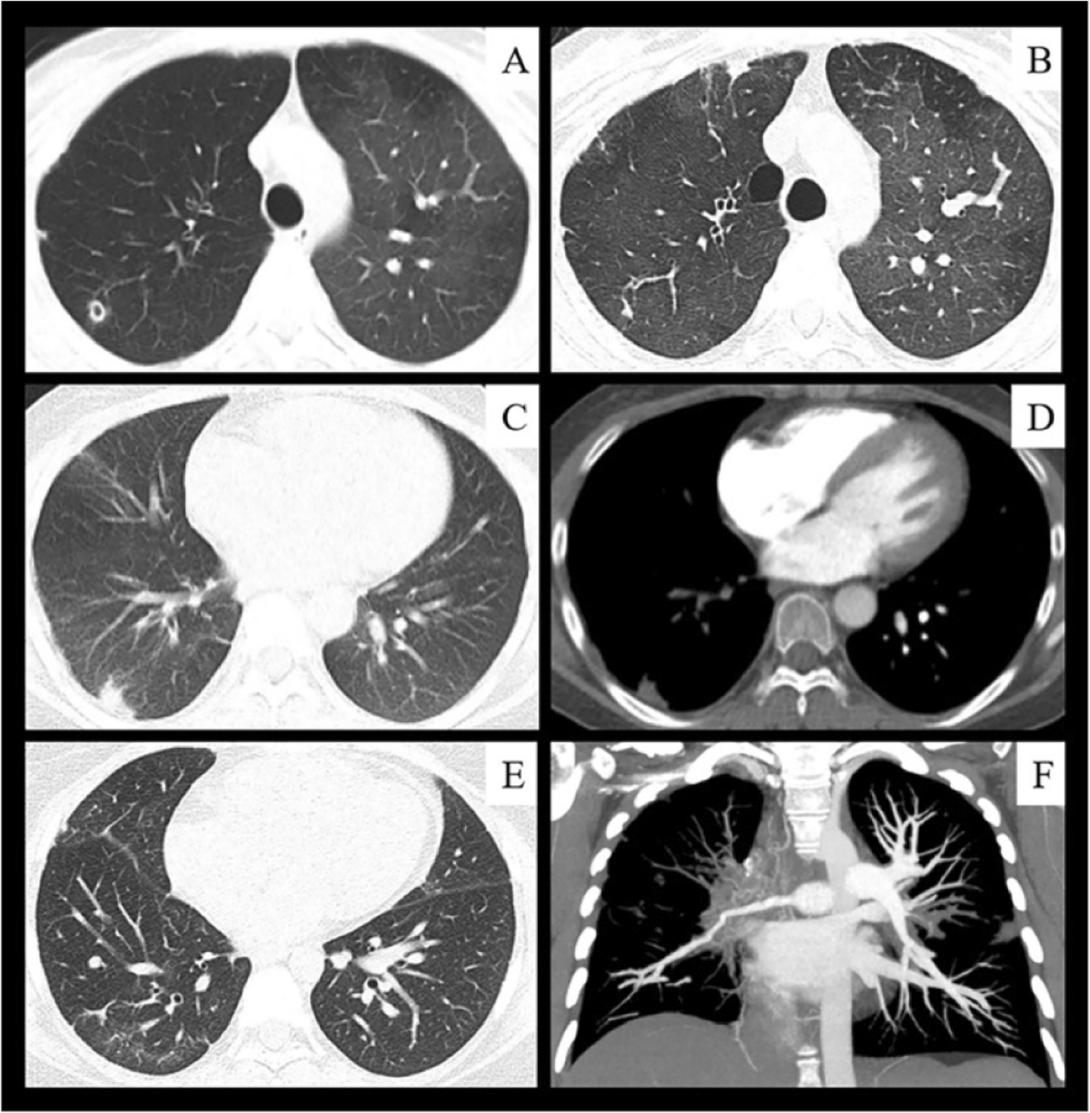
Imaging findings of pulmonary arteritis in patients with Takayasu’s arteritis. a, Axial computed tomography (CT) image shows mosaic perfusion with reduced vessels in the darker lung (right lung), indicating occlusive vascular disease; a thin wall cavity is present in the right upper lung, consistent with pulmonary infarction. b, CT image obtained 2 years later shows a healing residual lesion from the cavity of the right upper lobe; subpleural scarring is also present. c, CT image shows a subpleural wedge-shaped opacity suggestive of pulmonary infarction. d, Contrast-enhanced CT image shows corresponding pulmonary artery occlusion in the right lower lobe. e, CT image shows peripheral scarring from previous infarcts in the right lower lung. f, Coronal reformatted image from CT pulmonary angiography in the same patient as in (e) shows occlusion of the right upper lobe artery and stenosis of the right interlobar artery and lower lobe artery
